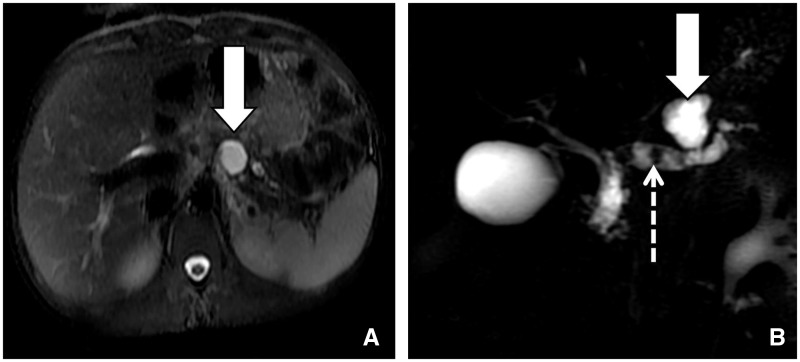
Pseudocyst in a 21-year-old male patient 5 weeks after acute interstitial pancreatitis. (A) Axial T2-weighted MR image, showing encapsulated small T2-hyperintense collection (arrow) with a well-defined wall. (B) Coronal 2D-MR cholangiopancreatography image showing dilated main pancreatic duct with intraductal calculi (dashed arrow) and ductal communication of the pseudocyst.
